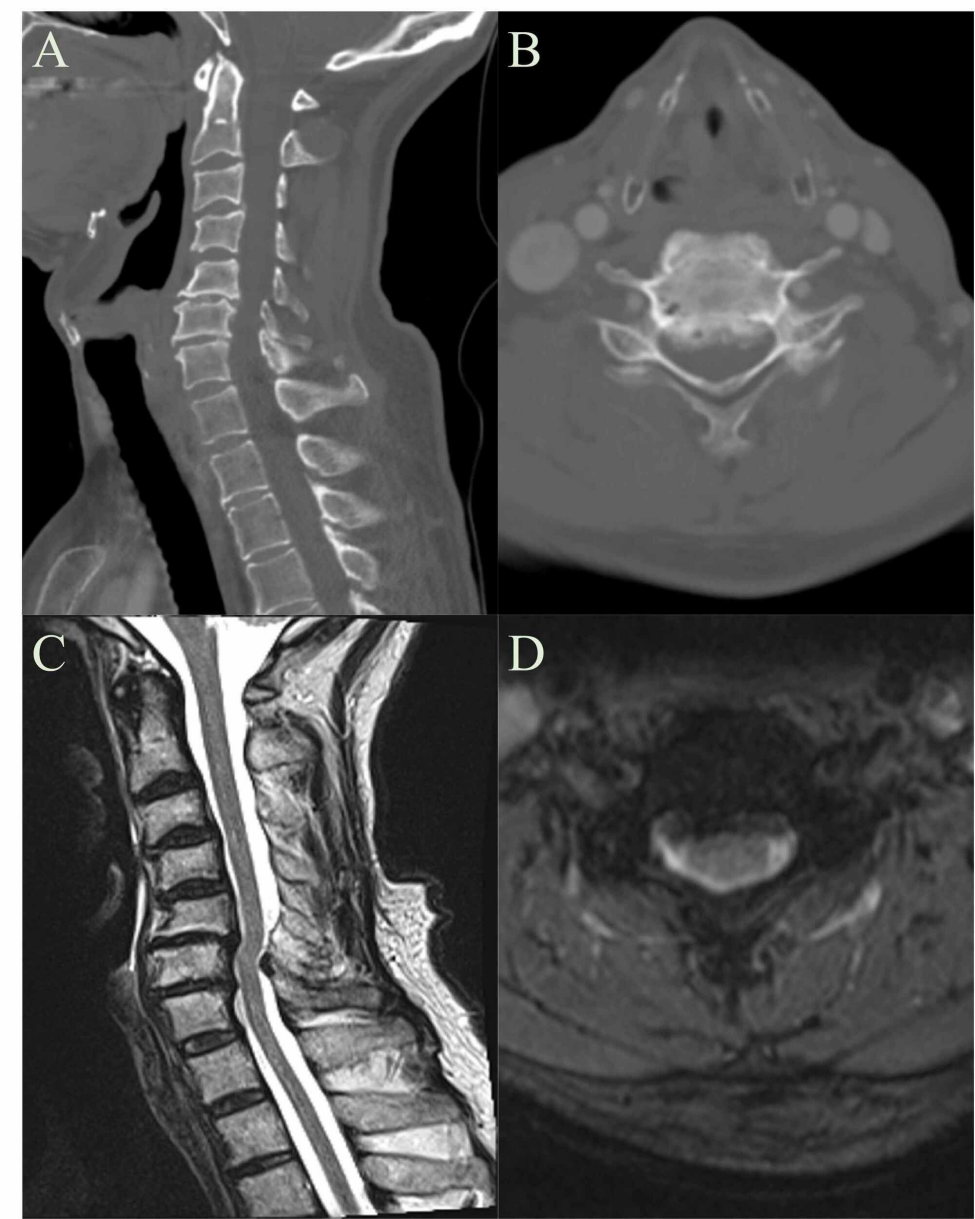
Patient 1 CT and MRI of the Neck A: Patient 1 CT Neck Sagittal View B: Patient 1 CT Neck Axial View C: Patient 1 MRI Neck Sagittal View D: Patient 1 MRI Neck Axial View Evidence of cervical stenosis is seen at C6-C7 (Cervical Vertebrae 6-7).
